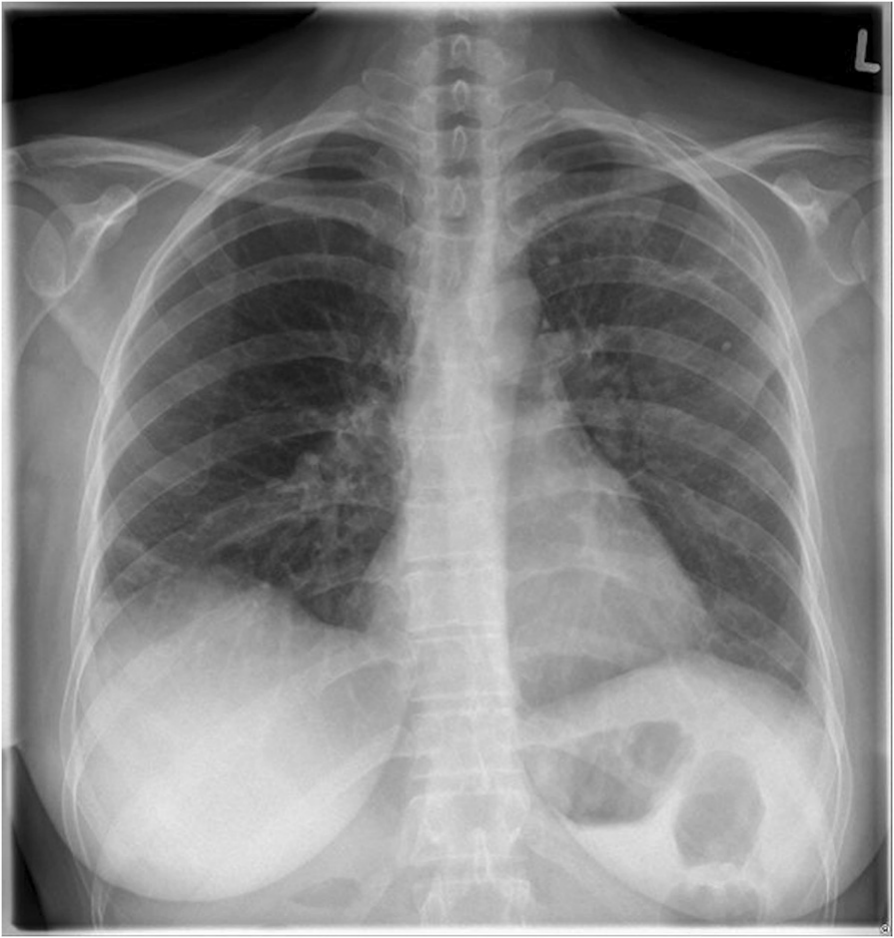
Standard chest radiography performed on the day of discharge from the hospital. Approximately one month after corticosteroid therapy, a dramatic improvement of pneumonic inflammation was observed with amelioration of bilateral infiltrates. Absence of pleural effusions.
